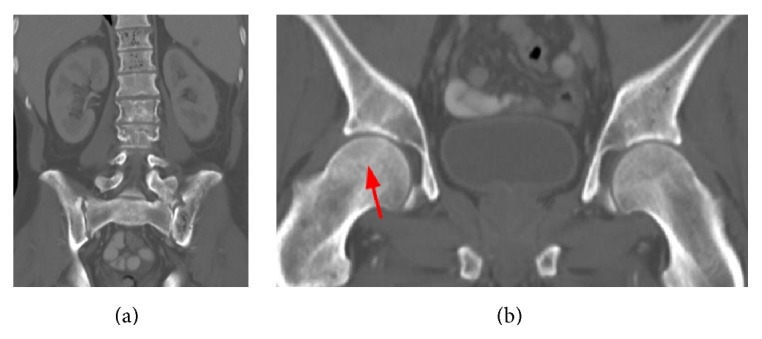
Coronal intravenous contrast enhanced CT images obtained 3 days after Figure 4. These images show (a) progression of osteonecrosis now involving the lumbar spine. (b) There is also subtle evidence of osteonecrosis of the right hip (arrow).
